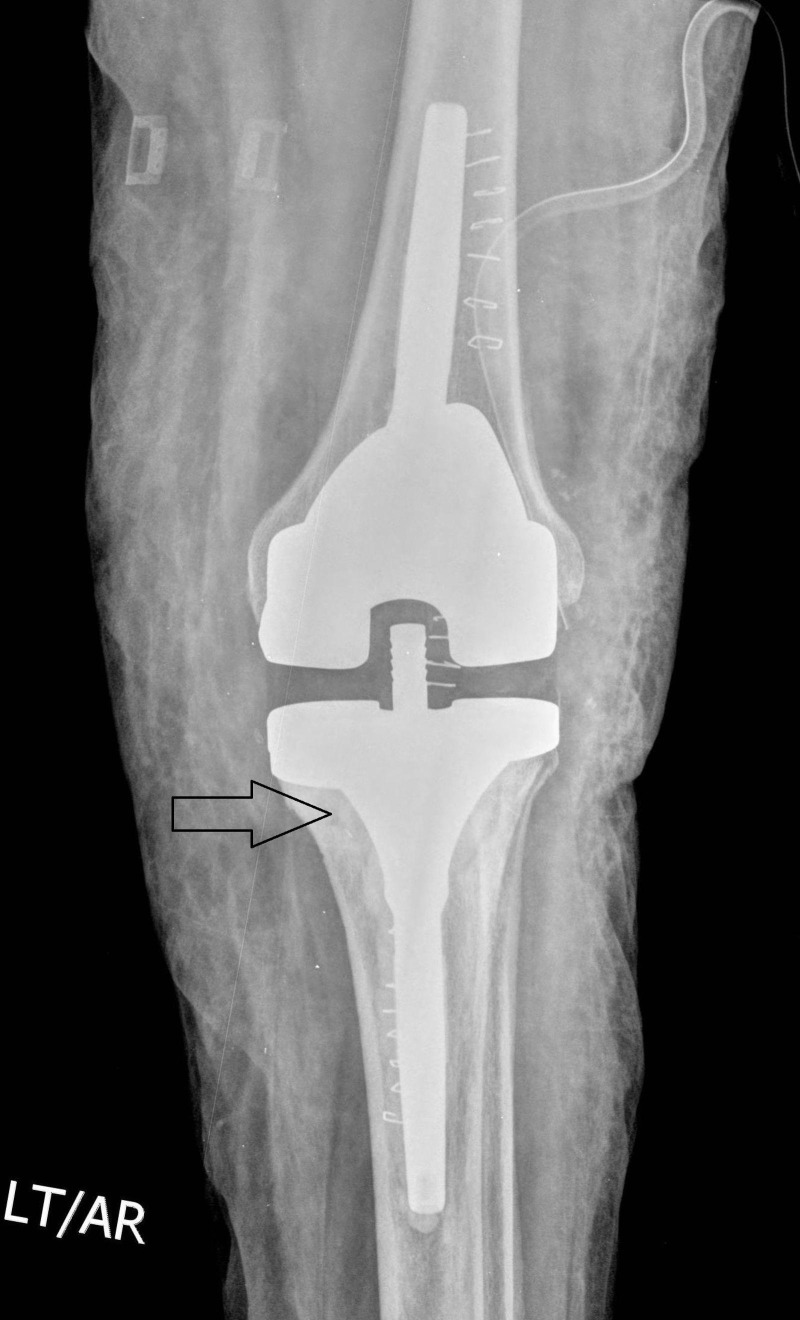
Postoperative X-ray (Anteroposterior View) Showing Revision Total Knee Arthroplasty (TKA)
